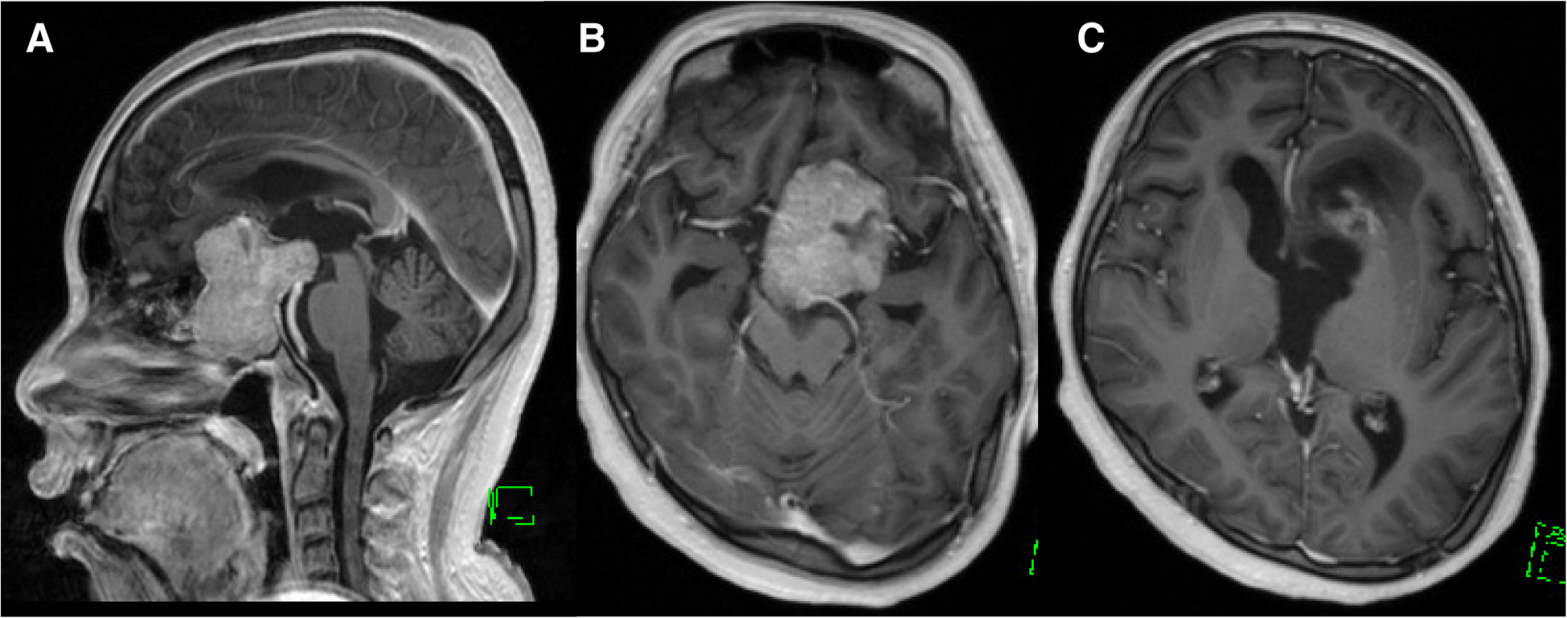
Gadolinium-enhanced T1-weighted images after dopamine agonist (DA) therapy. a Sagittal view. b and c Axial images. Tumor reduced significantly after the initiation of DA therapy with cabergoline
